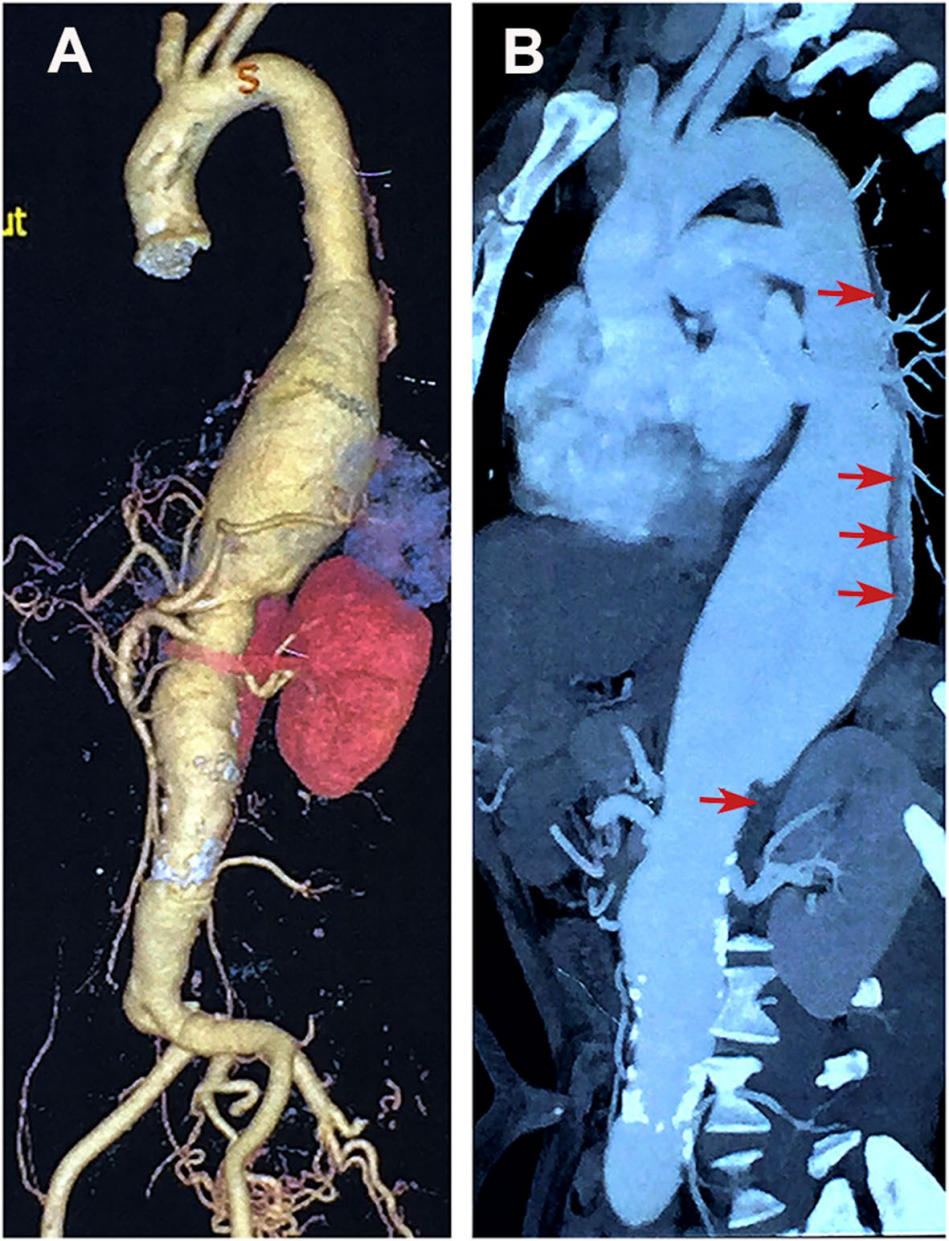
Preoperative reconstructed computed tomography images of the patient (A) CTA with 3D reconstructions revealing a type III thoracoabdominal aortic aneurysm (TAAA). B 2D sagittal view of the extent III TAAA with aortic intramural hematoma (red arrow)
